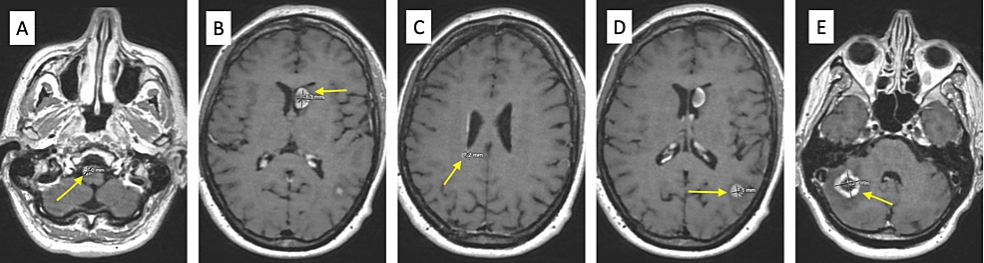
August 2023 MRI brain showing metastases in the A) right medulla, B) left thalamus, C) right periventricular white matter, D) left parieto-occipital region, and E) right cerebellum. Yellow arrows in each figure point to metastases.
